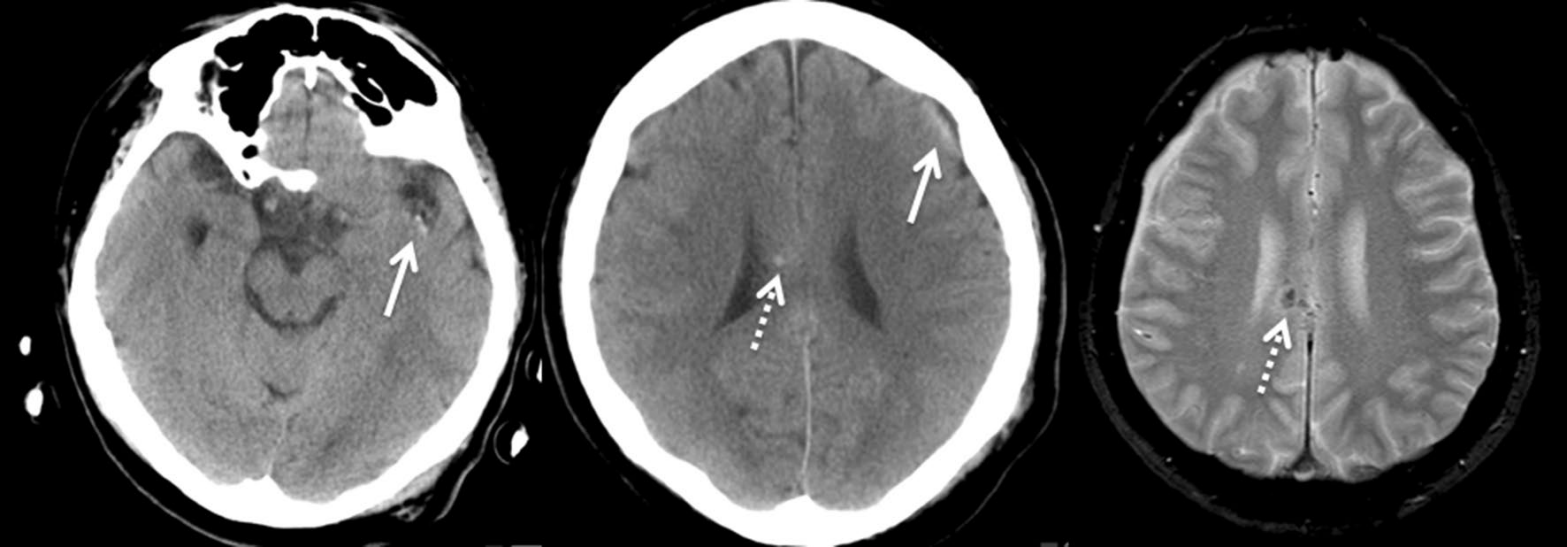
A 42-year-old man who was hit by a car while riding a bicycle. His GCS on admission to the emergency room was 14 out of 15. Two hours after admission, the patient’s GCS remained the same. The patient showed any of the New Orleans guideline’s items, therefore, his New Orleans score was 0. This is in contrast to the Canadian guideline, in which the patient had a GCS score of less than 15 at 2 h after admission (scored +1) and his mechanism of accident (car versus bicycle) fits the dangerous mechanism item (scored +1), leading to a Canadian Score of 2. On CT, the patients shows acute subarachnoid hemorrhages in the left Sylvian fissure (*left panel*, *arrow*) and on the surface of the left frontal lobe (*middle*, *arrow*). More importantly, some hyperdense foci that are suspected to represent a diffuse axonal injury are seen in the corpus callosum (*middle panel*, *dashed arrow*). The follow-up MRI study performed later, confirms the presence of DAI lesions on T2*WI (*right panel*, *dashed arrow*)
